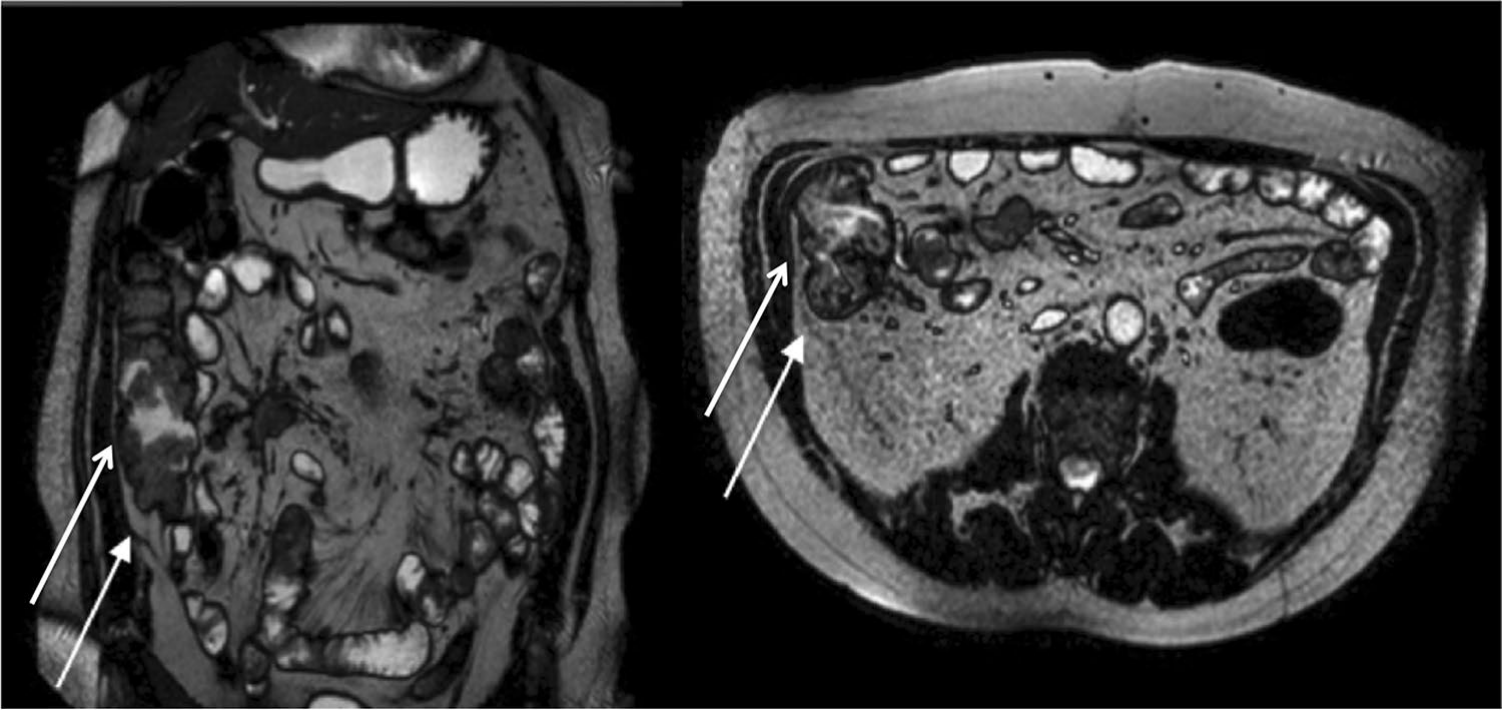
Pre-operative MRI shows a nodule within the wall of the distal ileal (arrow) and within the mesentery. Coronal (left) and axial plane (right)
